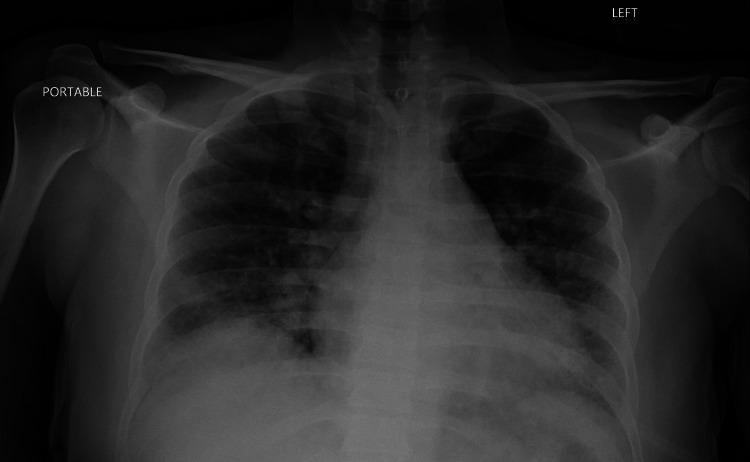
Anterior-posterior chest X-ray showing no significant change in diffuse bilateral patchy opacities compared to the initial chest radiograph on presentation
